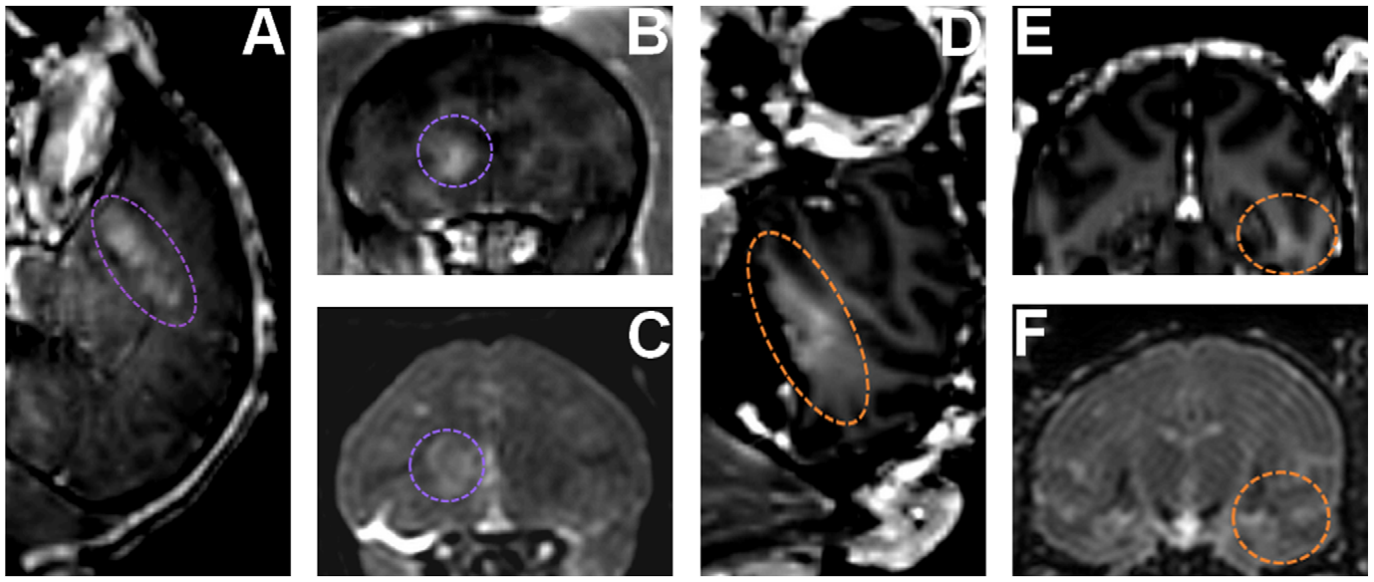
BBB opening in caudate and hippocampus & damage assessment. (A,B,C) BBB opening experiment targeting caudate using customized microbubbles and applying 0.6 MPa (purple dashed line shows region of interest). (D,E,F) BBB opening experiment targeting hippocampus using Definity® microbubbles and applying 0.6 MPa (orange dashed line shows region of interest). (A,B,D,E) 3D Spoiled Gradient-Echo (SPGR) T1-weighted sequence was applied after intravenous (IV) injection of gadodiamide 1 h after sonication. (A,D) Sagittal slices at the region of interest. (B,E) Corresponding coronal slices. (C,F) 3D T2-weighted sequence, an edema was visible using customized microbubbles while no damage was detected using Definity® microbubbles.
